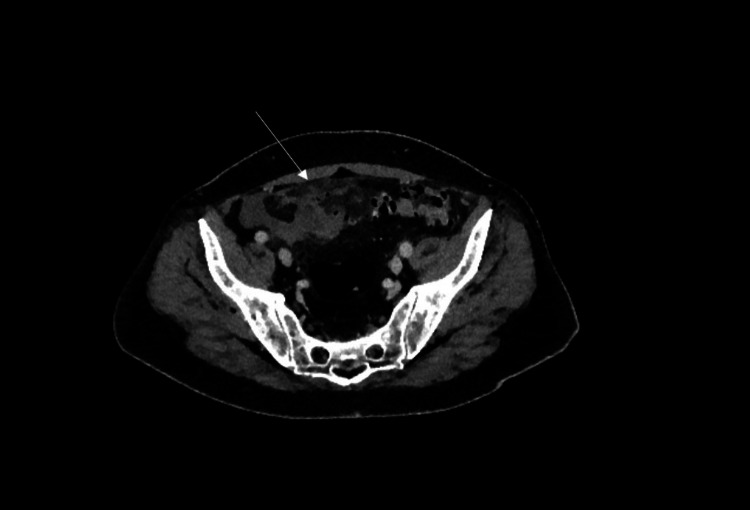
CT abdomen/pelvis showing significant inflammatory stranding surrounding diverticula of the sigmoid colon with pockets of free extra-luminal air.
